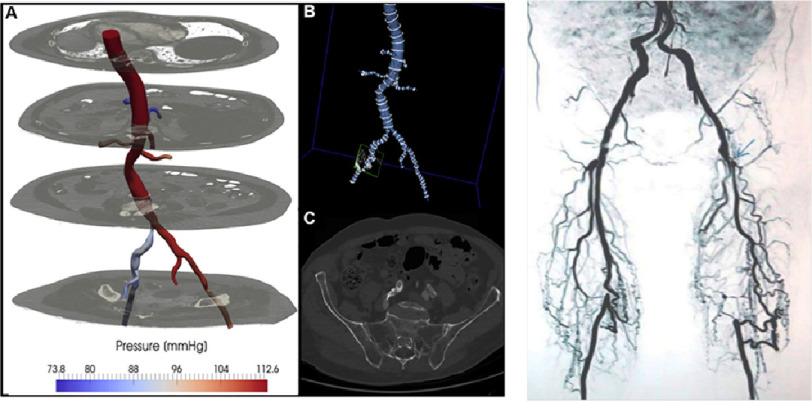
CT-based FFR computation of aortoiliac stenosis (left)^59^ and collateral vessel network around common femoral artery stenoses (right)^62^.
